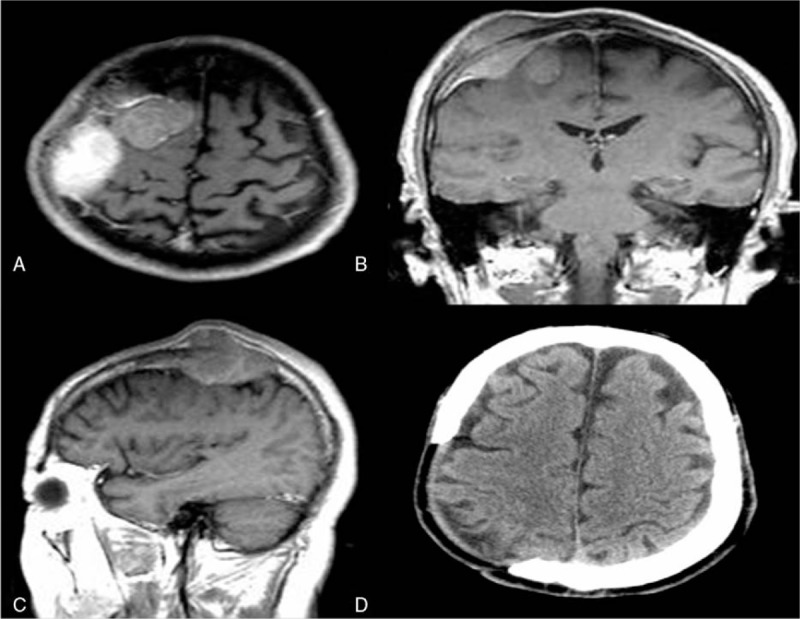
Preoperative axial (A), coronal (B), and sagittal (C) T1-weighted magnetic resonance images show 2 adjacent meningiomas in the right parietal lobe. Precontrast computed tomography scan taken on the fifth month after operation (D).
